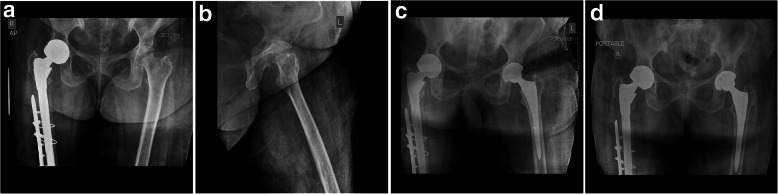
Pelvis and hips on X-rays. a an anteroposterior view preoperatively (left); follow-up X-rays after 2 years (uncemented ASR with S-ROM; right); b preoperative lateral view of left hip; c an anteroposterior view immediately after surgery (left) and follow-up after 2 years (right); d anteroposterior view at the final follow-up of 15.7 years (Uncemented ASR with S-ROM; right) and left hip (13.7 years; Pinnacle with Summit)
